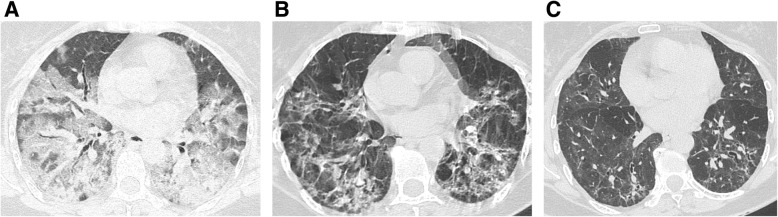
Computed tomography (CT) scans of the chest. a Initial CT scan showing bilateral ground glass opacities involving most of the lung parenchyma. b Follow-up CT scan 14 days later showing marked improvement from baseline. c Follow-up CT scan at 6 months
